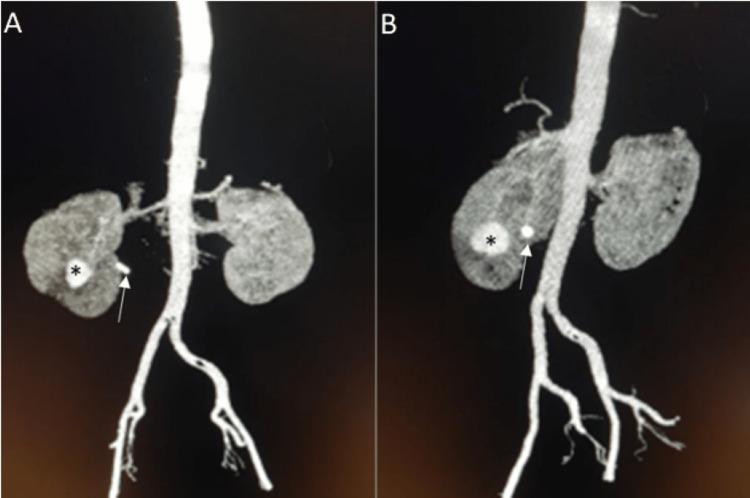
A two-week follow-up multidetector CT, coronal plane maximum intensity projection reconstruction demonstrates renal arteriovenous fistula (arrow) with associated pseudoaneurysm
